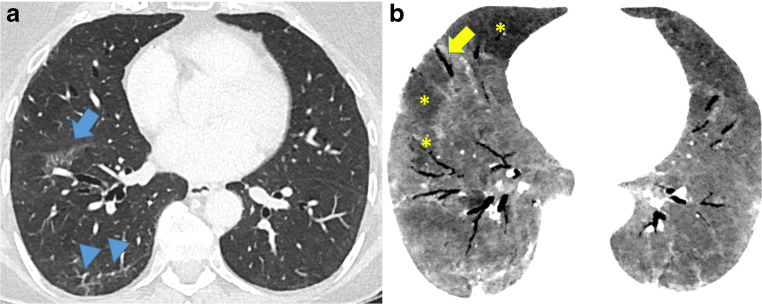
Axial CT section at the level of the lower lobes in a patient 4 months after severe COVID-19 pneumonia. Focal, subpleural reticulations with associated volume loss (image a, arrow) and subpleural bands (arrowheads in a) represent residual fibrous foci. Axial minimal intensity projections (10-mm slice thickness; image b) picture peripheral traction bronchiectasis. In addition, a common finding in post-COVID-19 patients is multifocal air-trapping (asterisk in b) pointing towards involvement of small airways
